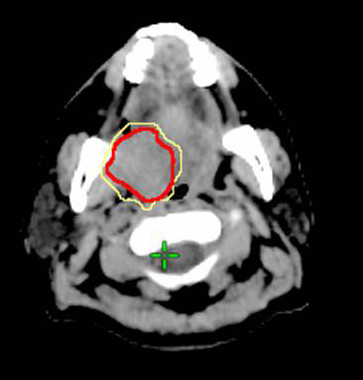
The biological target volume (BTV) of the primary tumor was determined when using 10% isodensity volumes (yellow line). CT-based GTV was outlined by red line.
